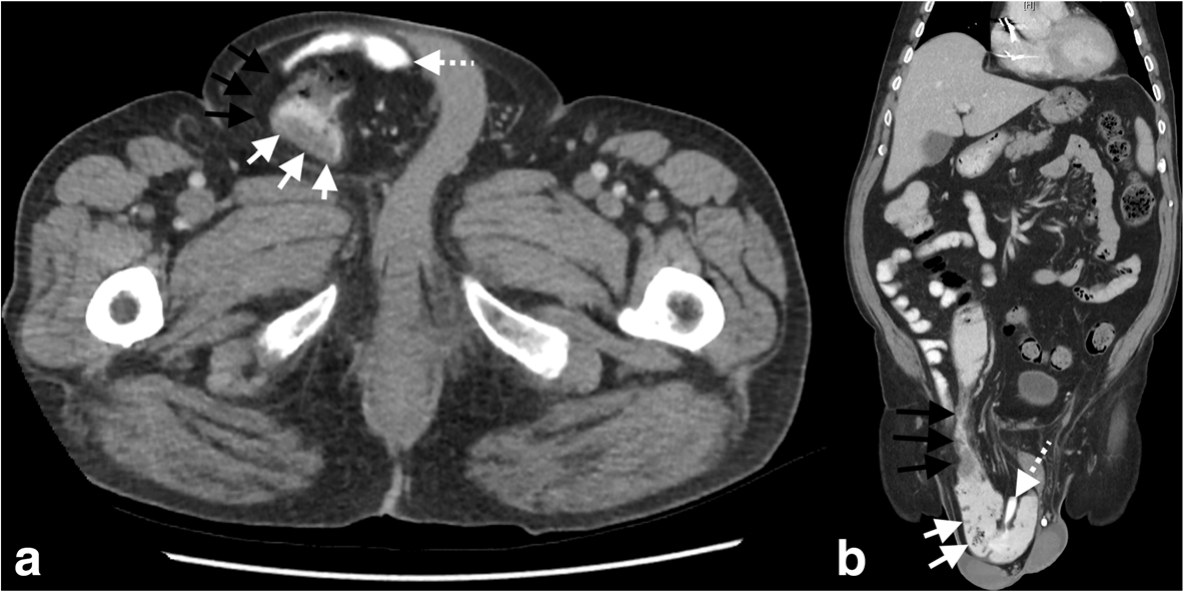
Computed tomography. a Axial view demonstrating right-sided inguinoscrotal hernia (black arrows) containing large bowel with thickening of the wall representing the ascending colon cancer (white arrows) and herniated terminal ileum (dashed white arrow). b Coronal view demonstrating the right-sided inguinoscrotal hernia (black arrows) with large bowel (white arrows) and terminal ileum (dashed white arrow) herniating through the inguinal canal
